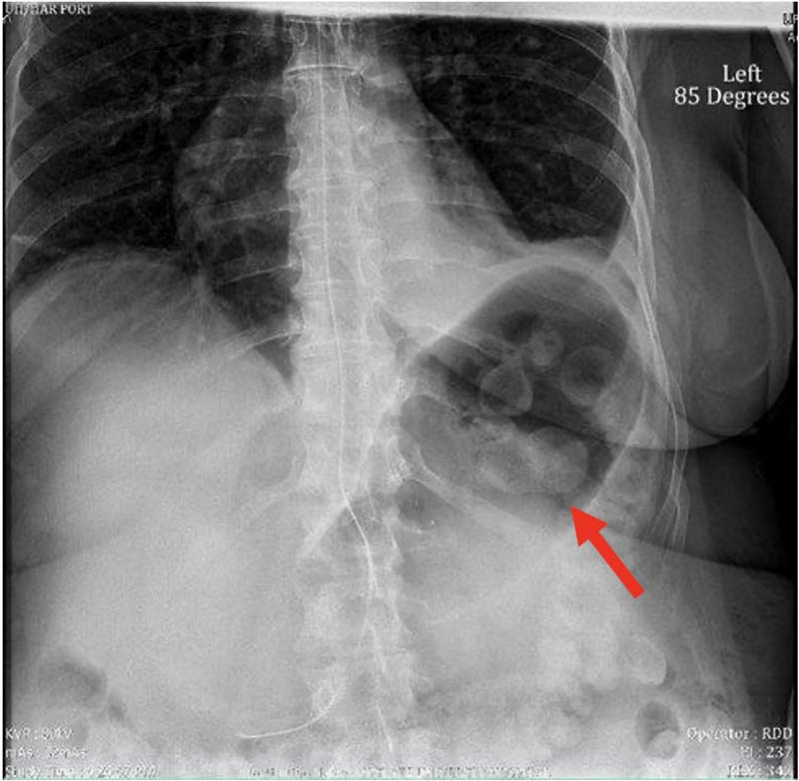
X-ray image of abdomen confirming persistent large gastric bubble despite adequately positioned NGT.
